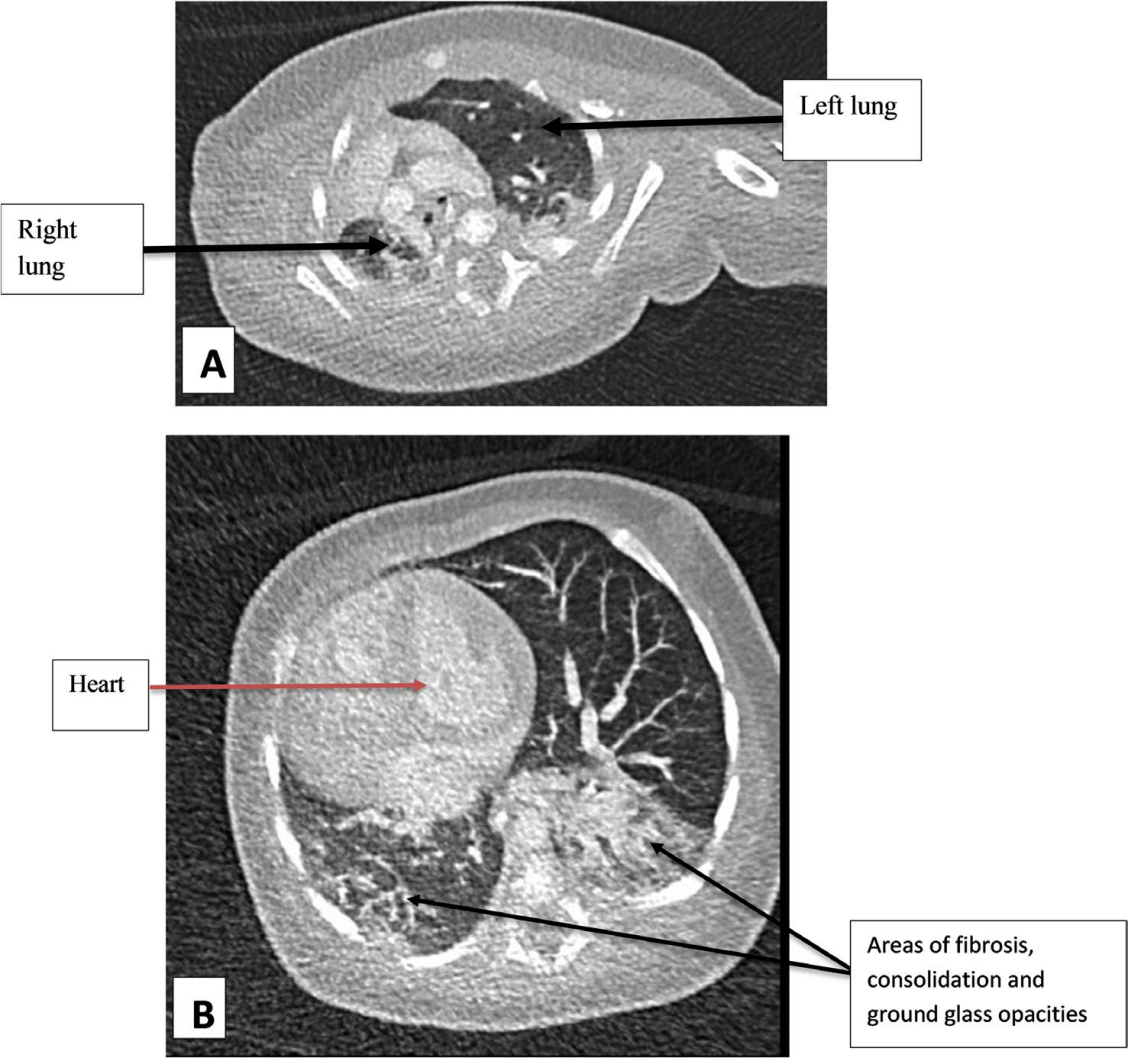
(A, B) Chest CT images in axial slices, lung window at the level of the lung apices and the ventricles respectively. The right lung is hypoplastic and the left lung is hyperinflated, crossing the midline. There are ground‐glass opacities, consolidations and fibrosis bilaterally, the right side worse than the left.
